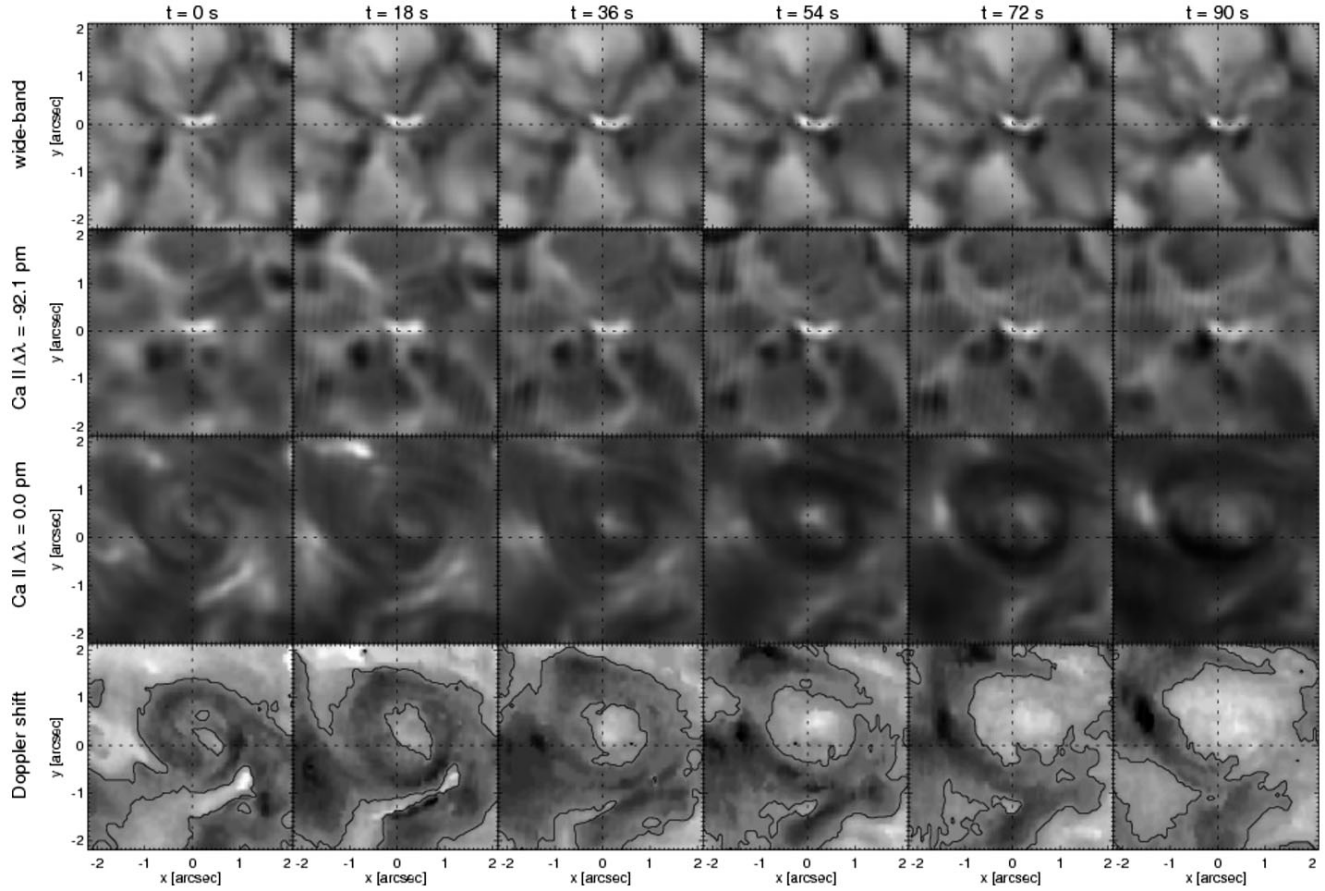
Snapshots from the temporal evolution (from left to right) of a chromospheric swirl seen in a wide-band filter centered on 854.2 nm, in narrow band filters of the Ca ii 854.2 nm line wing and the Ca ii line core, and of the Doppler shift (top to bottom rows, respectively). Black contours in the Doppler shift images indicate a zero Doppler shift value with grey scale shifts ranging from $-5.5~\text{km}\,\text{s}^{-1}$ (upflows) to $+5.5~\text{km}\,\text{s}^{-1}$ (downflows). Credit: Wedemeyer-Böhm and Rouppe van der Voort (2009), reproduced with permission ©ESO
